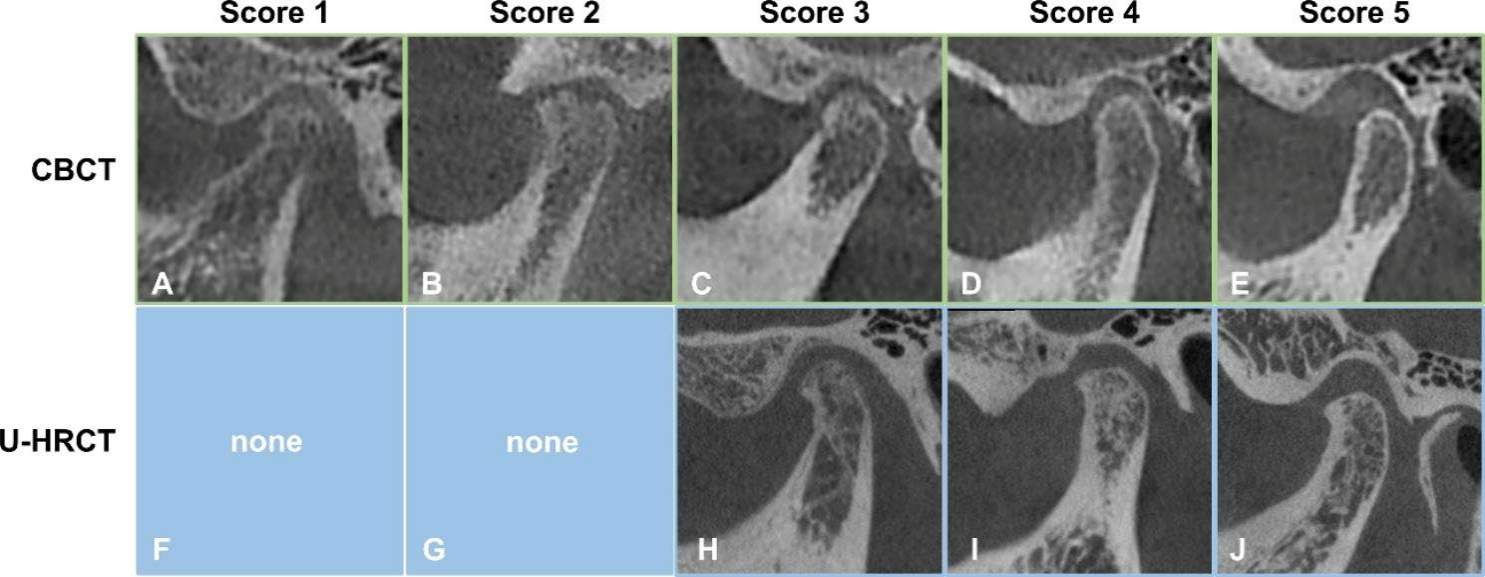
Image quality scores of CBCT and U-HRCT. Score 1 = unacceptable, nondiagnostic image quality (A); Score 2 = poor image quality, limited diagnostic value (B); Score 3 = fair, diagnostic image quality with mild artifacts or noise (C and H); Score 4 = good, diagnostic image quality to detect osseous changes (D and I); and Score 5 = excellent diagnostic image quality without artifacts or noise, optimal to make a diagnosis (E and J). Note that no cases are scored 1–2 for U-HRCT (F and G)
